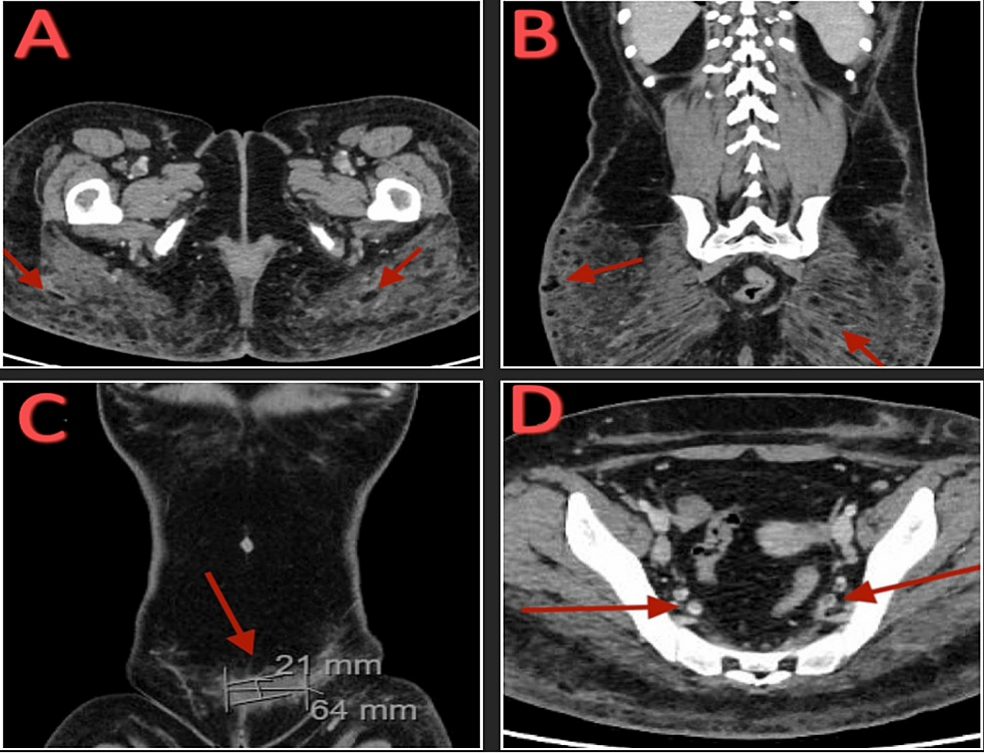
CT abdomen and pelvis with contrast. (A) Transverse view of small subcutaneous fluid collections and diffuse subcutaneous thickening and fat stranding in bilateral gluteal soft tissues. (B) Coronal view of small subcutaneous fluid collections and diffuse subcutaneous thickening and fat stranding in bilateral gluteal soft tissues. (C) Coronal view of a small subcutaneous fluid collection measuring 21 mm × 64 mm. (D) Filling defect in bilateral common femoral veins.
